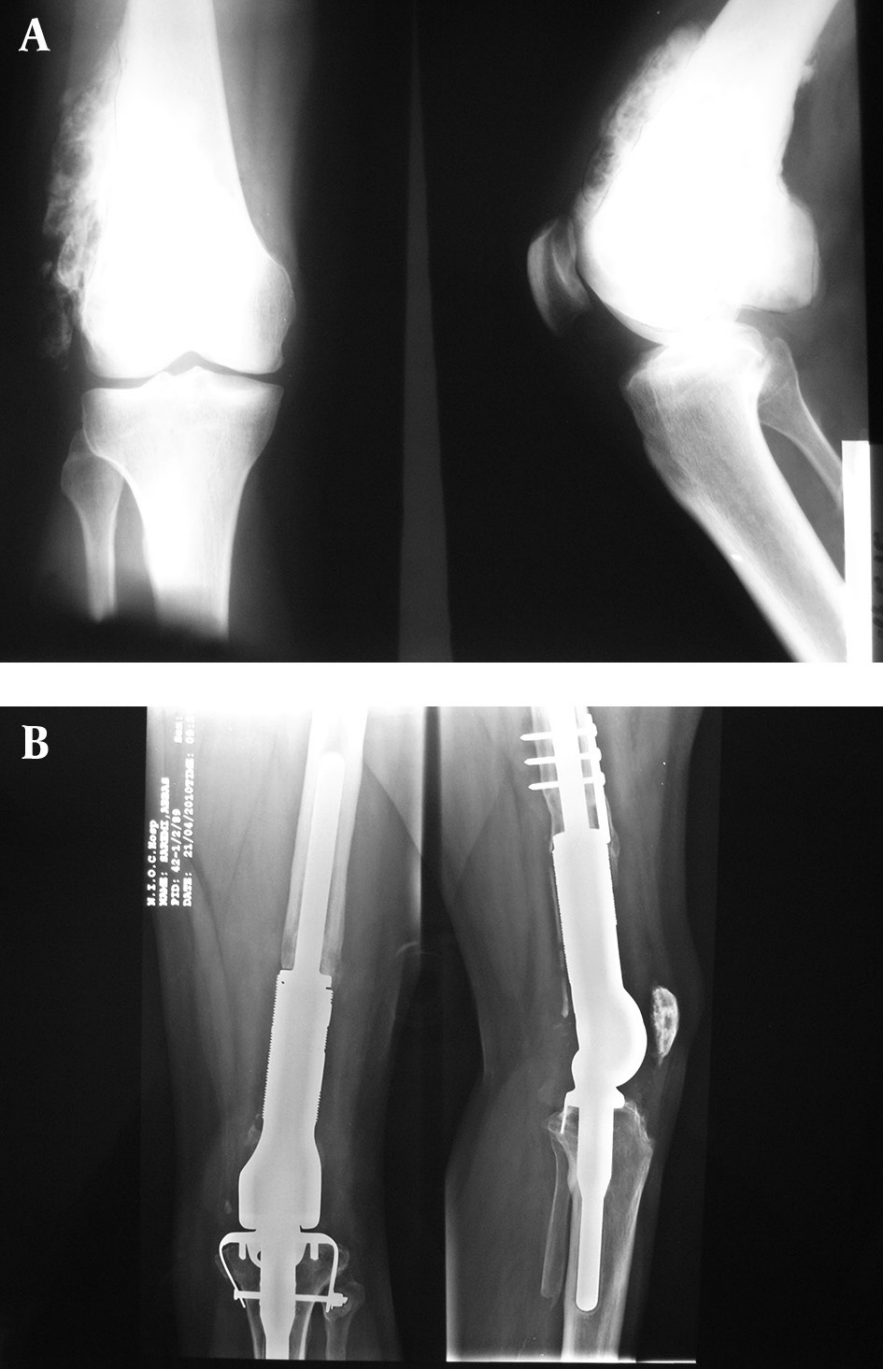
Anteroposterior and Lateral X-rays of the Left Knee Showing Distal Femur Parosteal Osteosarcoma (A). Post Total Knee Arthroplasty X-rays After Knee Fusion (B).
